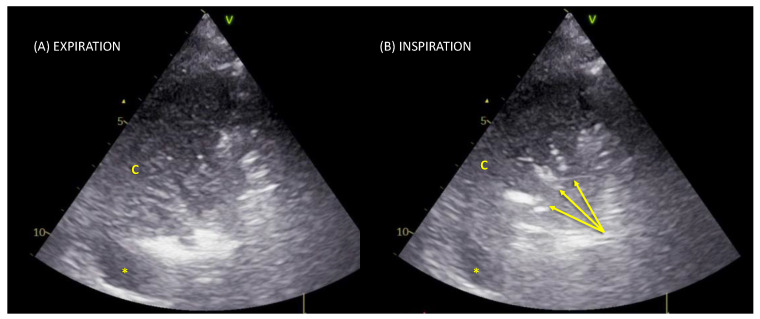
Lung ultrasound scan of a typical linear–arborescent air bronchogram. Posterior region in the transversal scan with a phased-array probe. Panel (A) in expiration, a tissue-like pattern corresponding to a consolidation (c) is visualized; a small pleural effusion is also visible as an anechoic space (*). Panel (B) during inspiration, a linear–arborescent air bronchogram appears (yellow arrows): a sign highly specific for ventilator-associated pneumonia.
